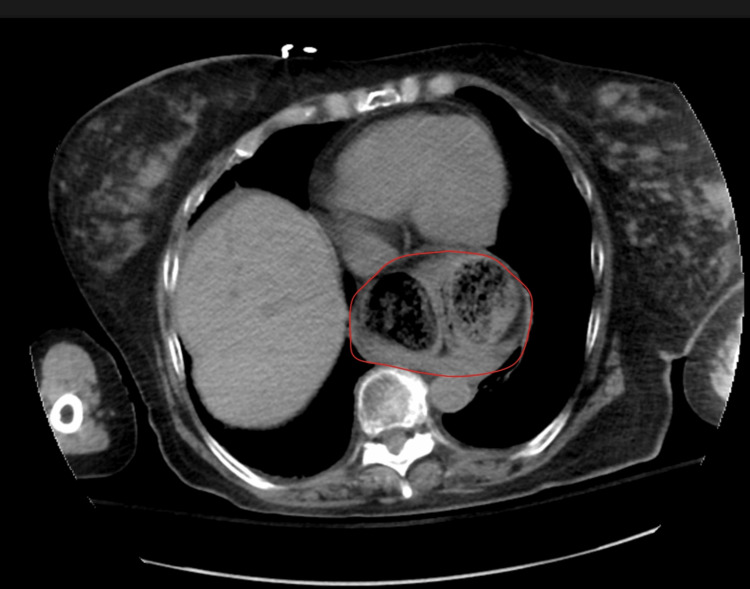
Transverse view of a CT abdomen and pelvis above the diaphragm level showing the apex of the sigmoid volvulus within the thoracic cavity, compressing the heart. The large bowel within the thorax is delineated in red. CT: computed tomography
